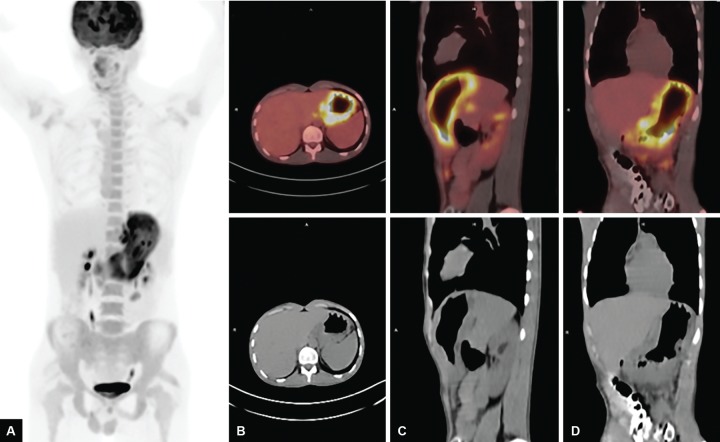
Maximum intensity projection (MIP), axial, sagittal, and coronal F-18 FDG PET/CT images before ATT; MIP image (A) demonstrated diffusely increased FDG uptake throughout the stomach. Axial (B), sagittal (C), and coronal (D) PET/ CT images showed intense FDG uptake in the thickened gastric wall, and intra-abdominal enlarged lymph nodes
